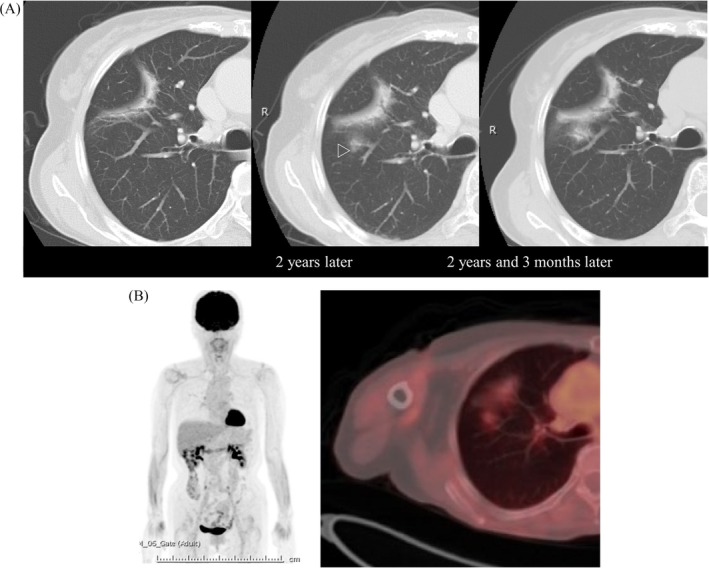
(A) Chest CT scan showing the appearance of a second lesion (▷) adjacent to the first lesion in the right upper lobe. Over time, both nodules increased in size and eventually merged, forming an area of radiological fusion. (B) PET‐CT demonstrating low fluorodeoxyglucose (FDG) uptake with SUV_max values of 1.6 for the first lesion and 1.79 for the second lesion.
